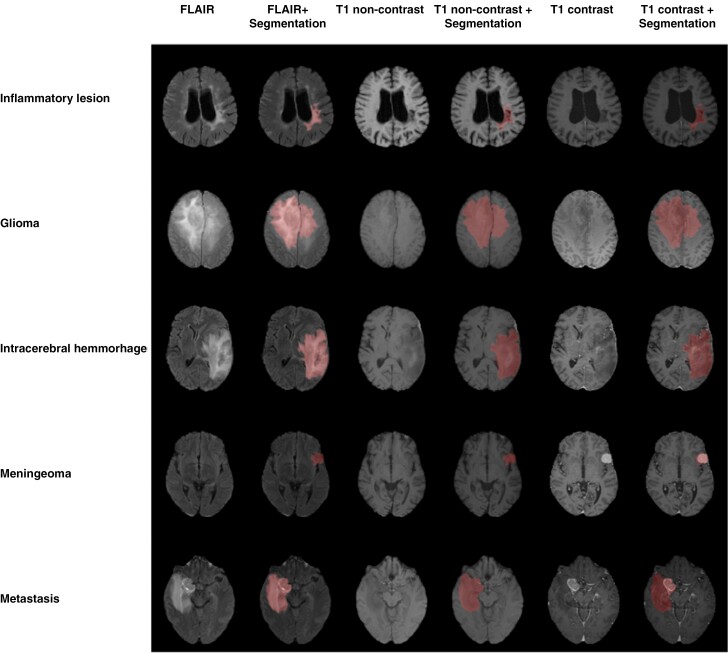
Examples of fully automated segmentations and their coregistration with the respective FLAIR, noncontrast T1-weighted sequence, and contrast-enhanced T1-weighted sequence.
